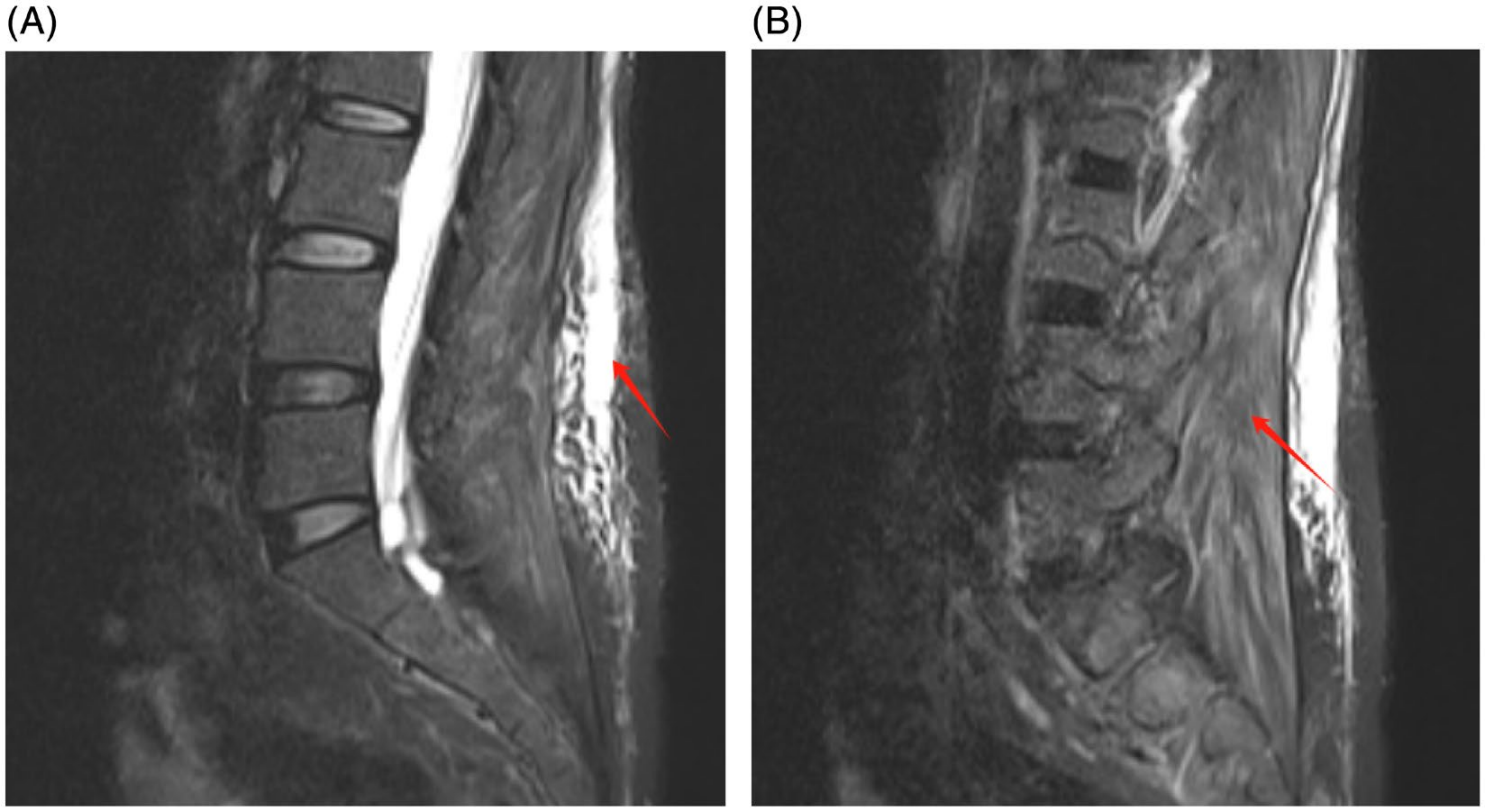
Axial T2‐weighted MRI of the lumbosacral region. (A) High‐signal edema in subcutaneous soft tissues (arrow). (B) Swelling of paraspinal muscles (arrow).
